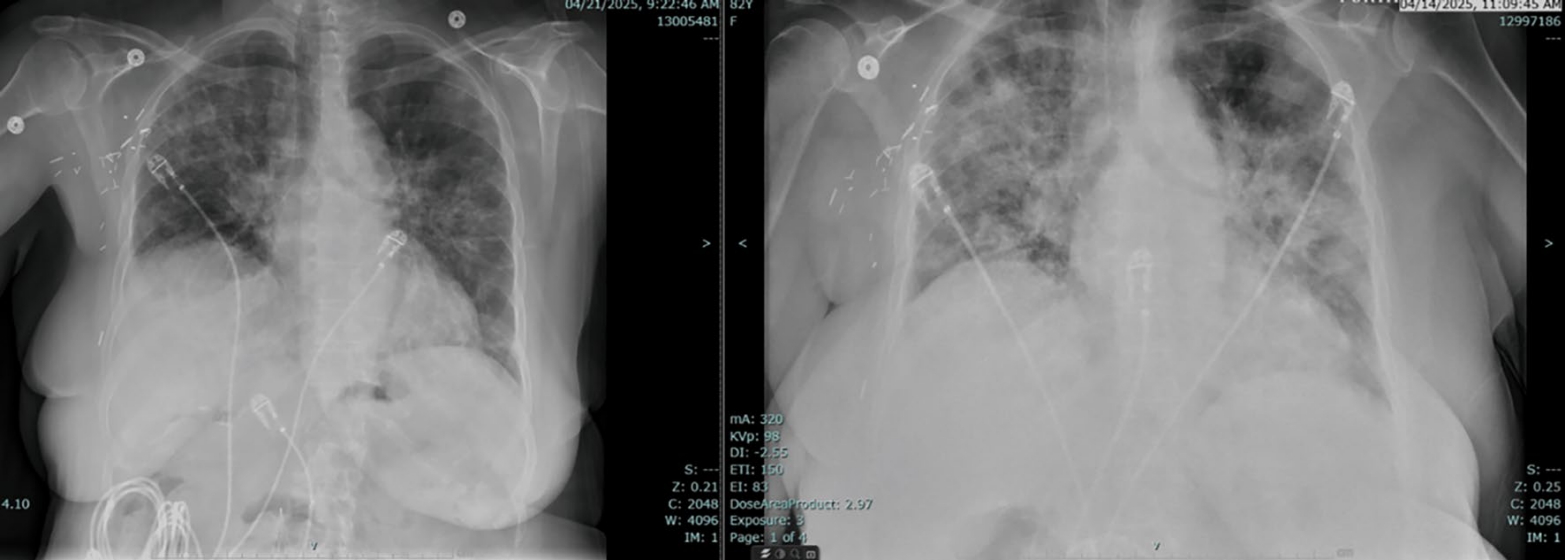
Follow‐up chest radiograph showing persistent diffuse opacities.
